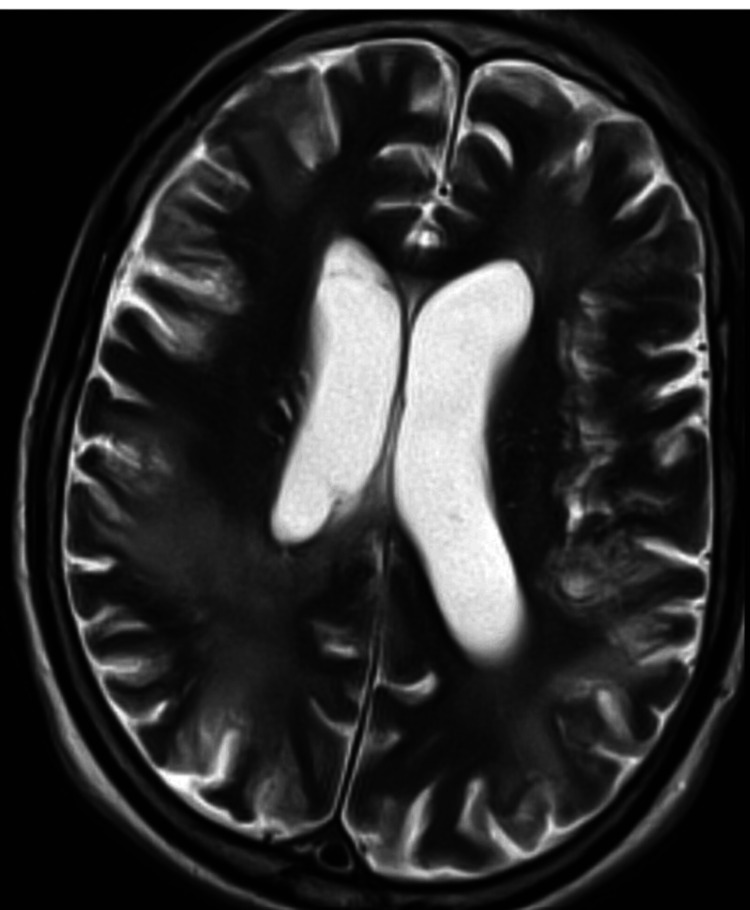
Interval MRI of the brain study showing significant improvement in the burden of the ring-enhancing brain lesions MRI: magnetic resonance imaging
